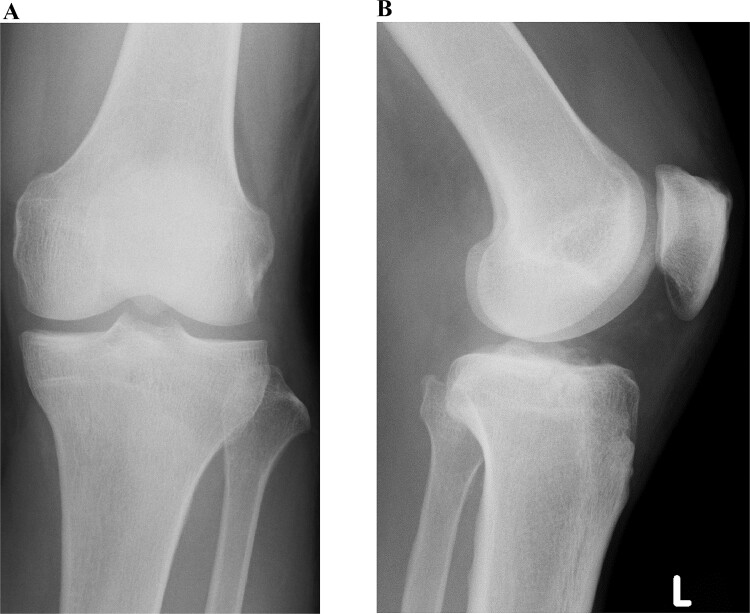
Preoperative plain radiographic images of the left knee. Articular depression of the tibia is shown on a lateral-view radiograph.
